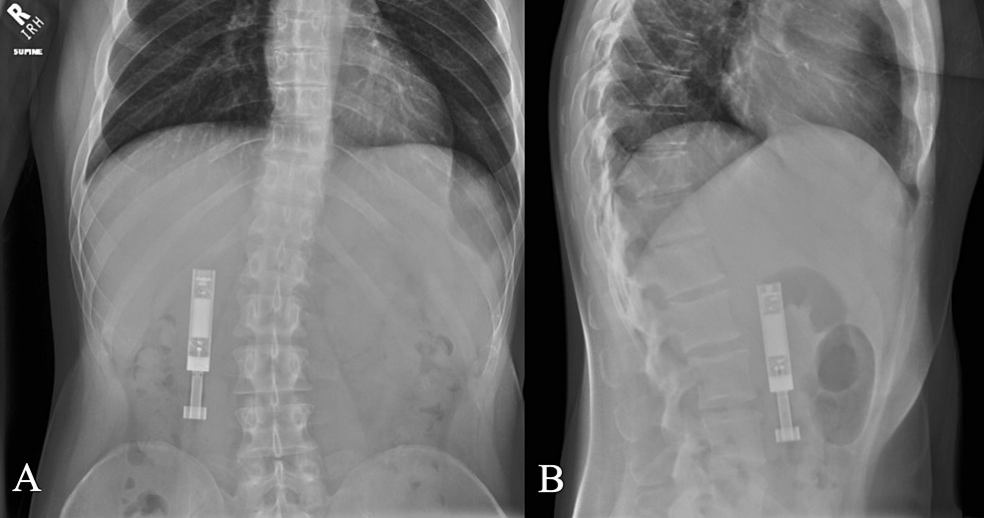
Abdominal plain film demonstrating an ingested vape pen. (A) Anterior-posterior, and (B) lateral.
